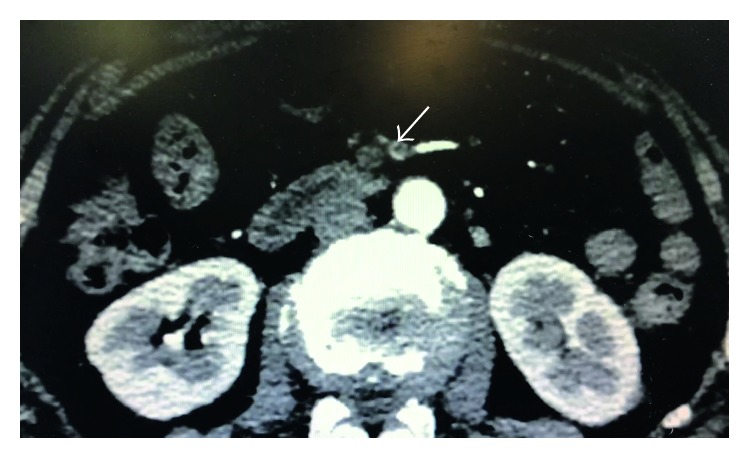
Abdominal computed tomography with contrast material showed occlusion of the superior mesenteric artery.
